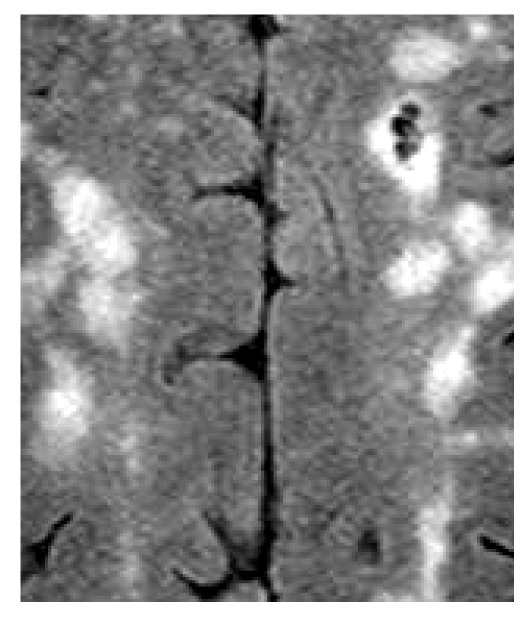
Enhancement of the brain MRI using contrast adjustment.
